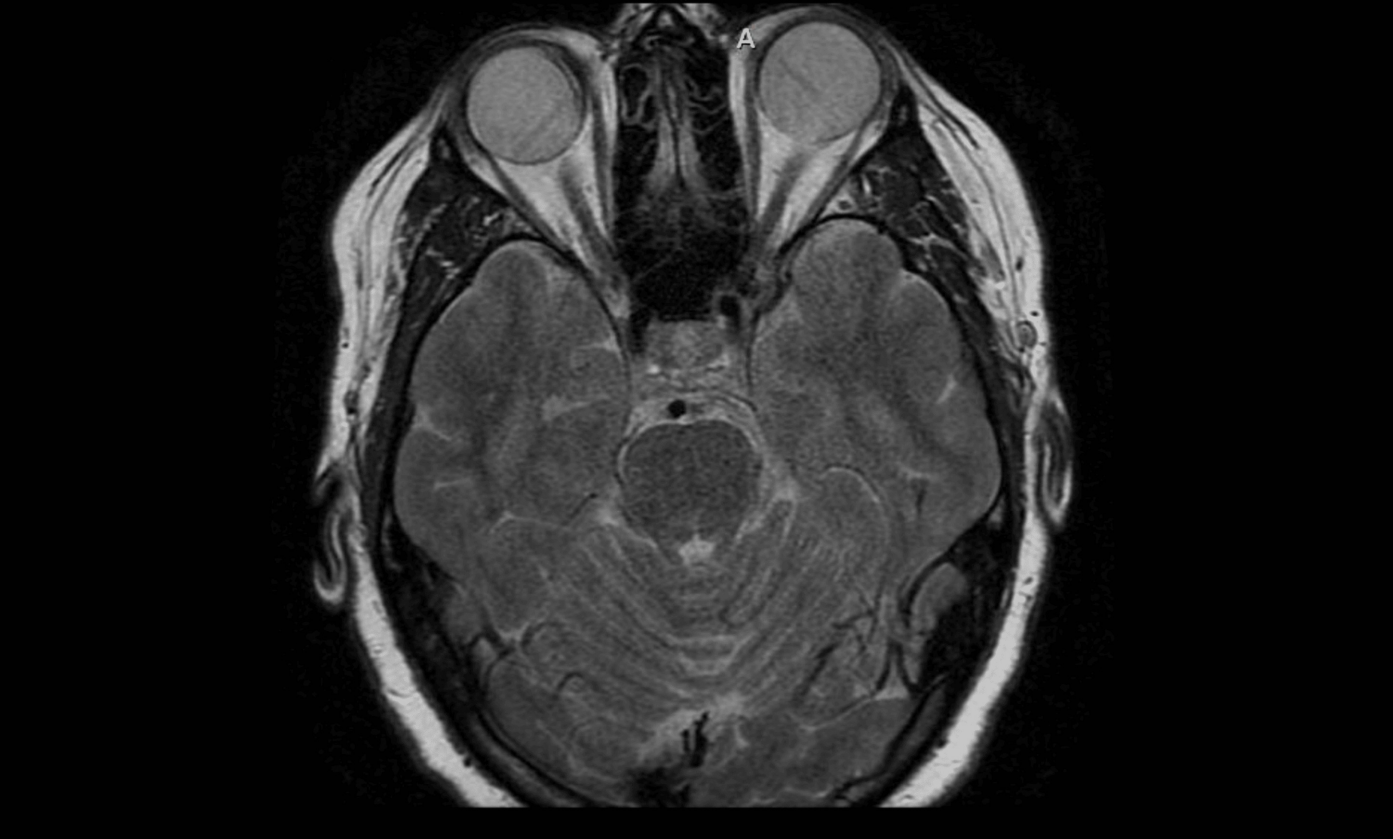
Magnetic resonance imaging (MRI) brain and orbits, T2 weighted, showing normal orbital and brain anatomy
